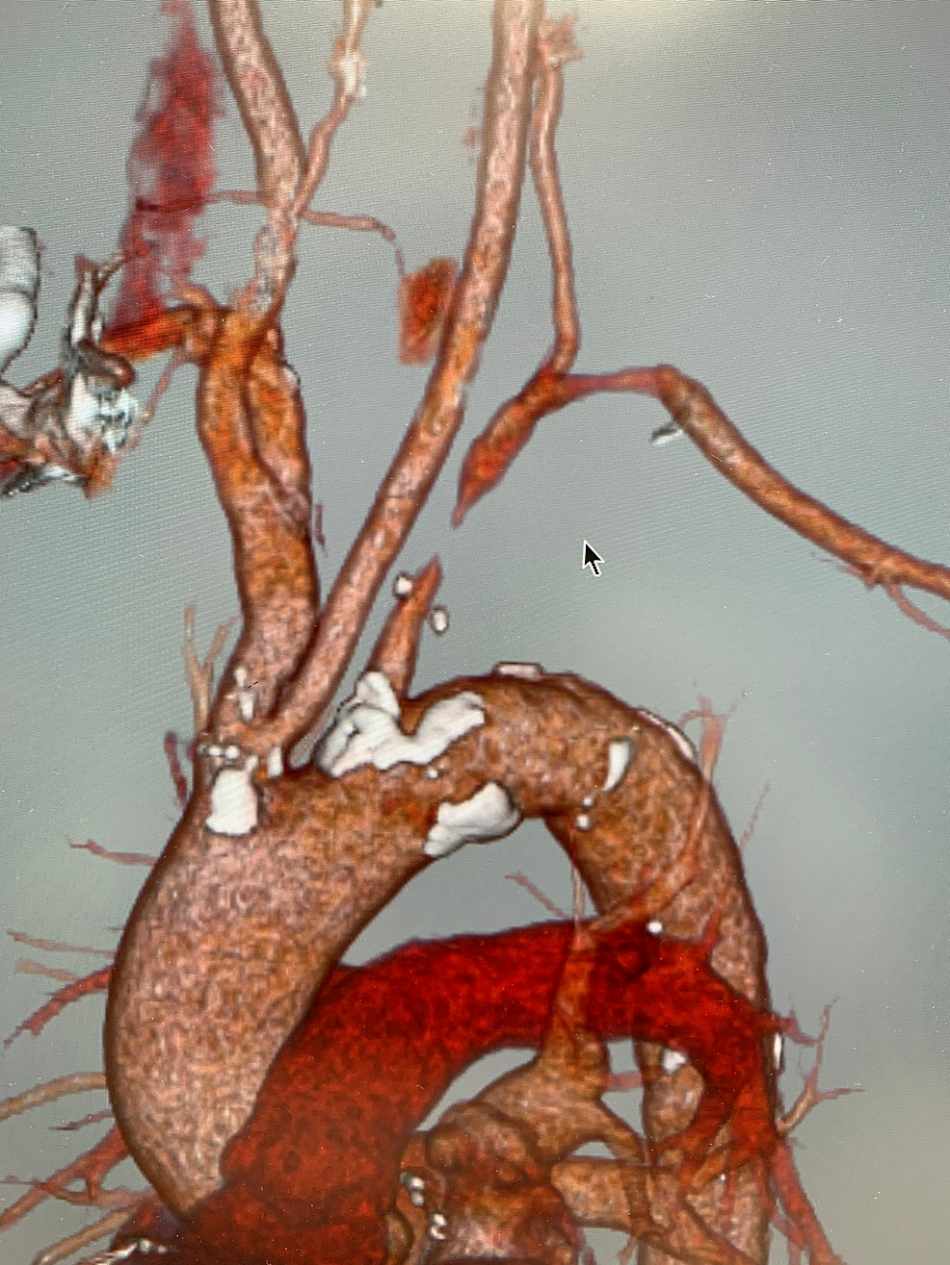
Contrast CT CT image revealed almost complete occlusion at the origin of the subclavian artery CT, computed tomography
